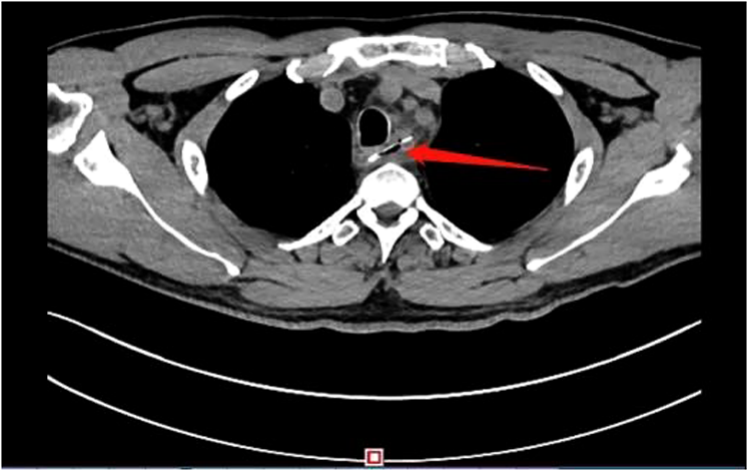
Chest CT showed a hyper-dense shadow of a fish bone shape in the upper Esophagus.
